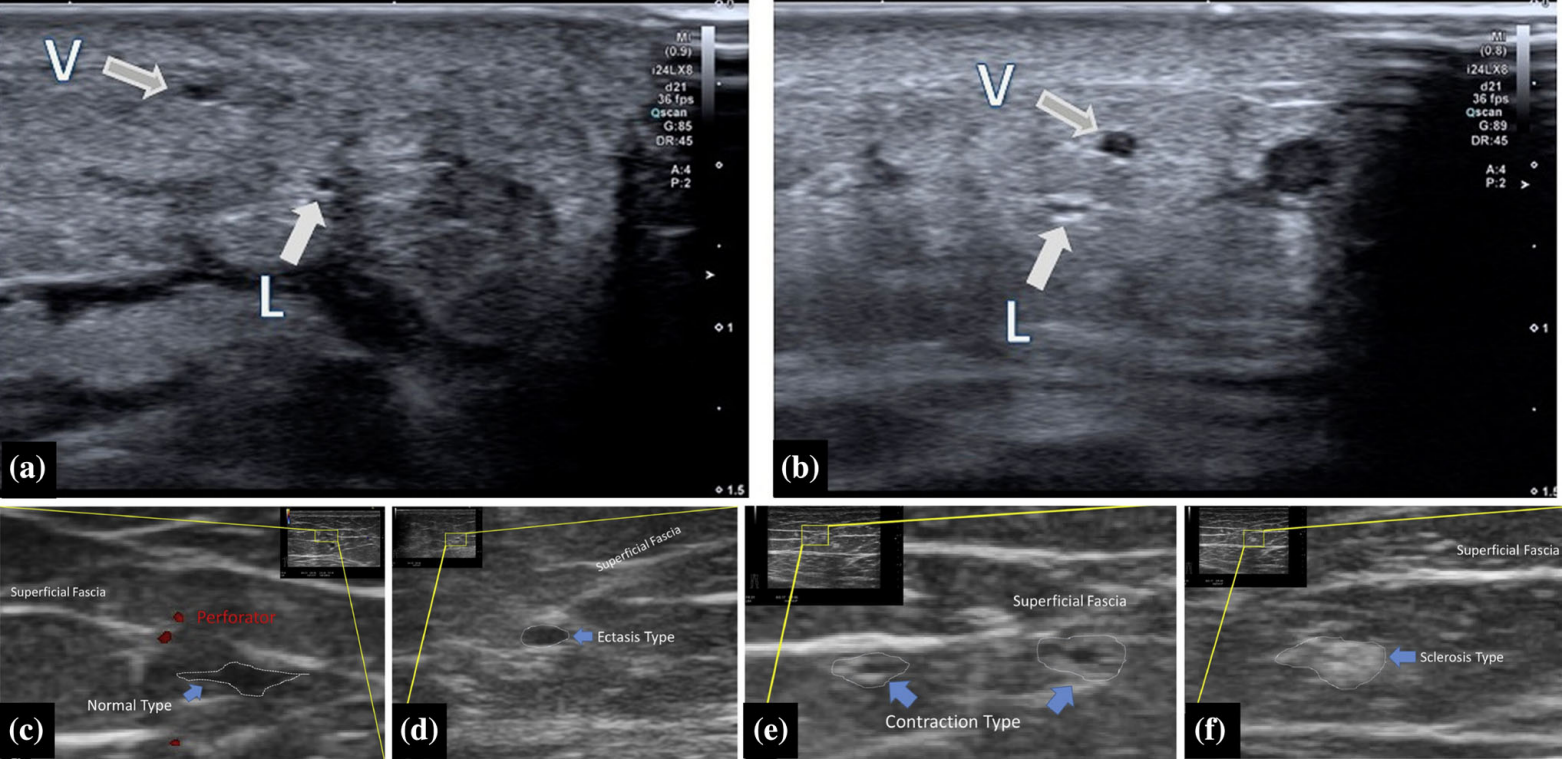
a, b Ultrasonographic images of veins (V) and lymphatic vessels (L). Reprinted from Czedik-Eysenberg et al.^137^ with permission from John Wiley & Sons, Inc. c, d, e, f Ultrasonographic images of different lymphatic vessel types according to the NECST classification. Reprinted from Mihara et al.^134^ with permission from Elsevier
